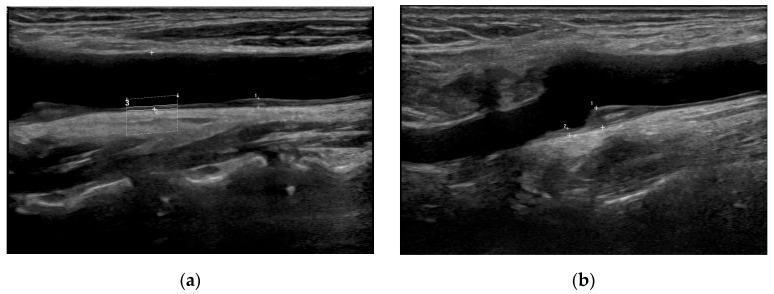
Representative carotid ultrasound images. (a) Grade I carotid plaque in the left common carotid artery. A small protuberant plaque is visualized with intima–media thickness (IMT) < 1.5 mm. (b) Grade III carotid plaque in the left internal carotid artery bulb. A large, protruding plaque with IMT ≥ 2.5 mm is noted, indicating high-grade carotid pathology.
